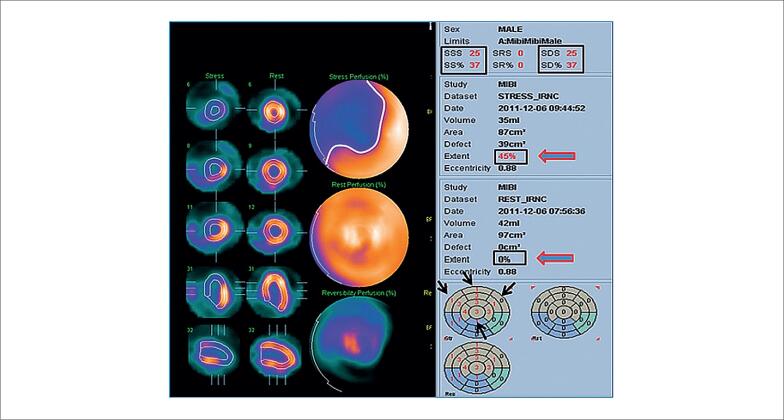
NA GATED-SPECT: isquemia estresse induzida de grande extensão. Nota-se extensa área de hipocaptação envolvendo as paredes anterior, região septal, ápice, estendendo-se, ainda, à porção distal das paredes inferior e lateral, evidenciada em coloração azul e delimitada no mapa polar (MP) de estresse por linha branca (imagem superior à esquerda). O MP representativo da perfusão em repouso evidencia distribuição homogênea do radiofármaco, traduzindo perfusão normal. A representação gráfica dos escores de estresse (setas pretas), evidencia a graduação numérica da intensidade da hipocaptação e o número de segmentos acometidos (canto inferior direito), com os somatórios SSS = 25, SRS = 0 e SDS – 25 (canto superior direito), além do porcentual de miocárdio em risco estimado em 45% do ventrículo esquerdo (carga isquêmica). A cinecoronariografia evidenciou lesão proximal suboclusiva de artéria descendente anterior. SDS: somatório da diferença dos escores (*summed difference score*); SRS: somatório do escore de repouso (*summed rest score*); SSS: somatório do escore de estresse (*summed stress score*).
